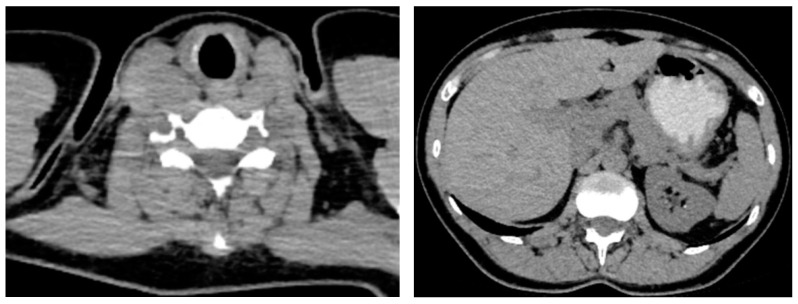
Intravenous contrast computed tomography (transversal plane): post-operatory aspect 12 years after total thyroidectomy for medullary thyroid carcinoma and bilateral adrenalectomy for bilateral pheochromocytoma: thyroid region without any aspects suggestive for remaining tissue (on the left); empty adrenal spaces without recurrence or remnants (on the right).
